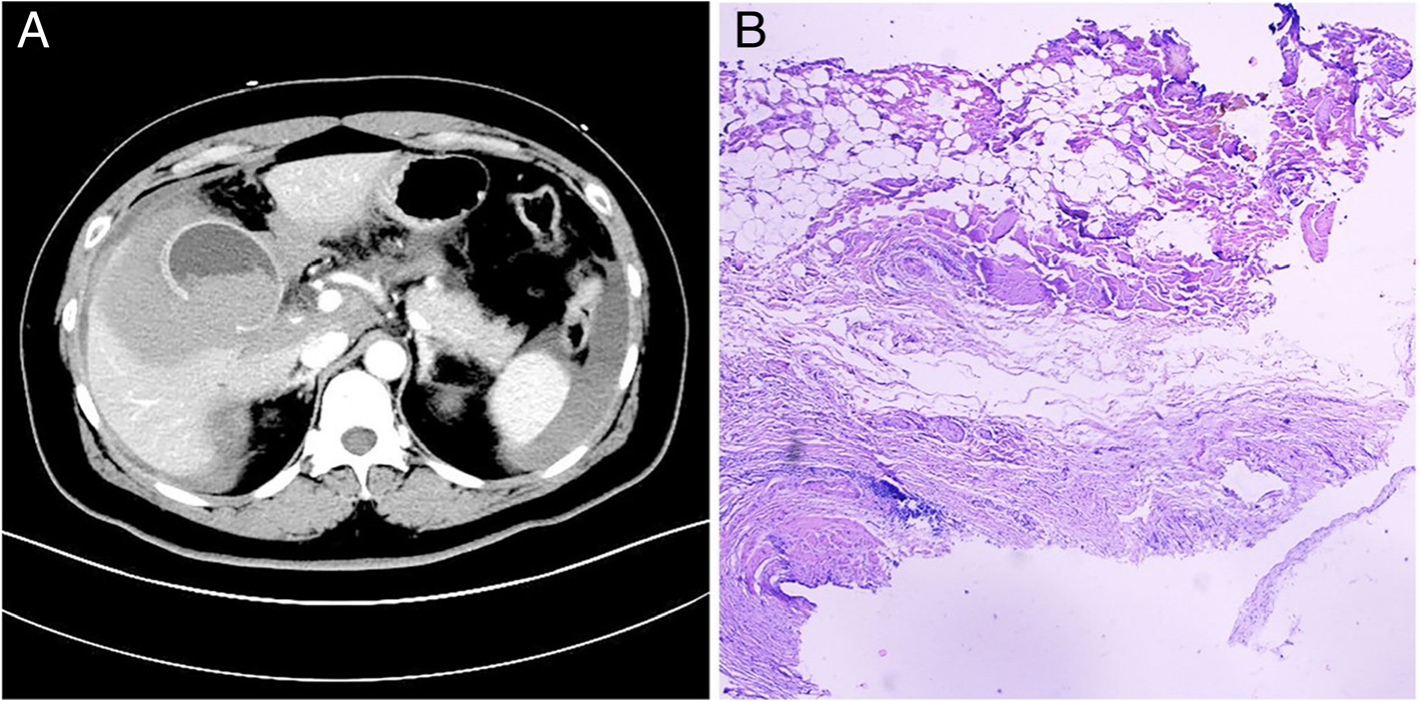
a, Abdominal CT scan with intravenous contrast showed a 2.5 cm filling defect in the body of gallbladder wall, a 1.0 × 0.8 cm stone in the neck of gallbladder and a massive hematocele in the abdominal cavity. b, Final pathology showed a disruption in the muscularis propria of partial gallbladder wall and plenty of eosinophils and lymphocytes infiltration in the mucous layer, associated with chronic cholecystitis
